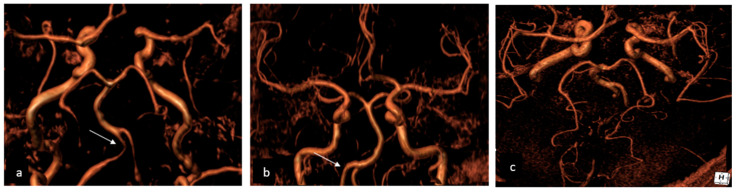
A 58-year-old female admitted to the Emergency Department due to thunderclap headache, diagnosed with RCVS. (a) The initial Angio CT showed bilateral V4 vasoconstriction (arrow) and narrowing posterior cerebral arteries; (b) three months later, the follow-up Angio CT showed complete resolution of vertebral artery vasoconstriction (arrows); (c) the follow-up imaging also showed complete recanalization of posterior cerebral arteries. RCVS: reversible cerebral vasoconstriction syndrome.
